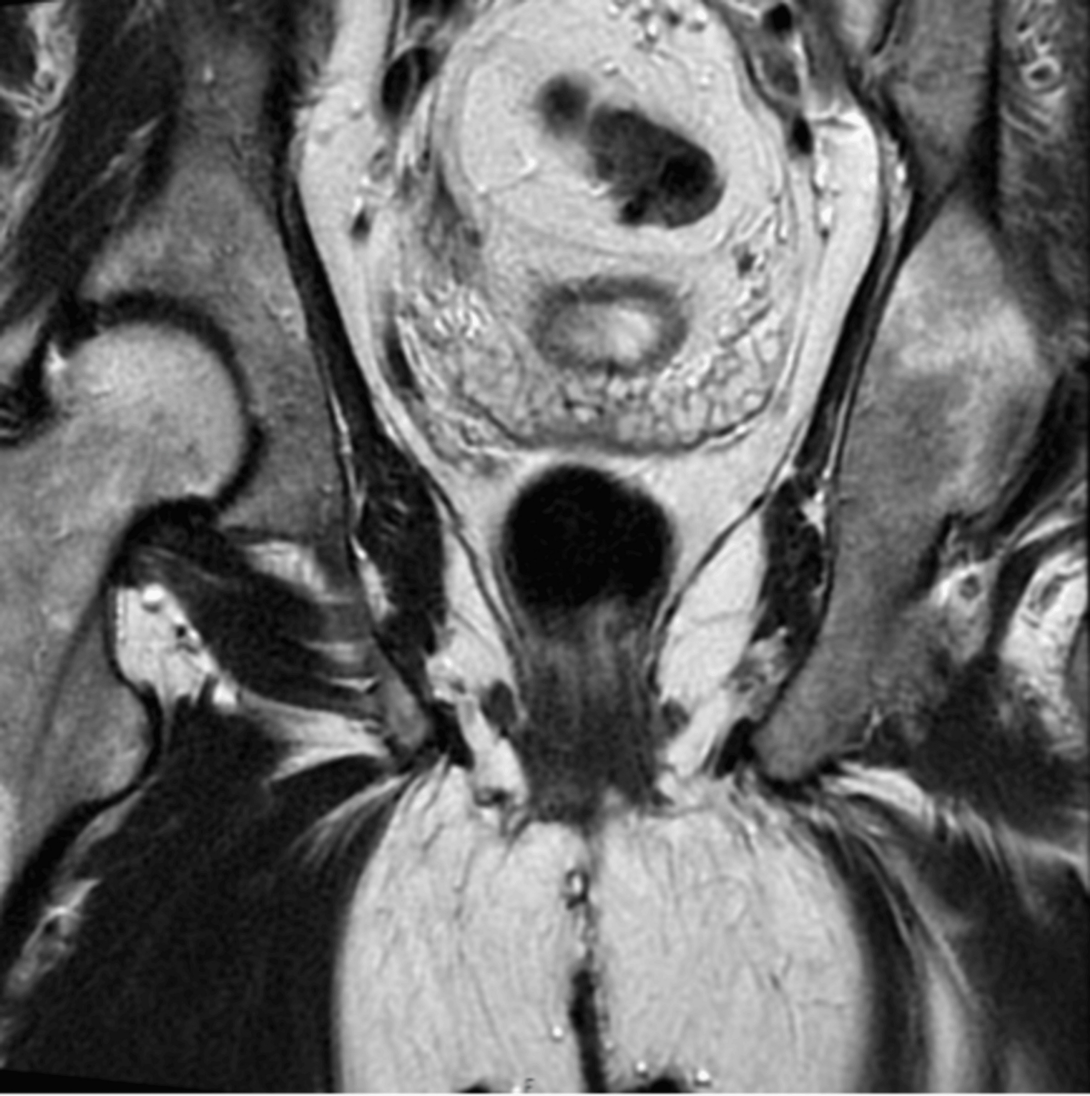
MRI image (coronal T2) showing enlarged right seminal vesicle with evidence of inflammation MRI, magnetic resonance imaging
